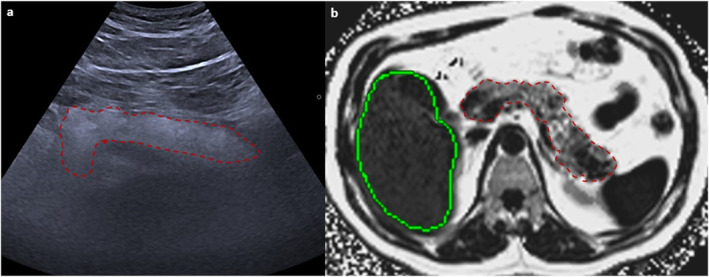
Transabdominal ultrasonography image (a) of a patient with fatty pancreas showing increased echogenicity, which was confirmed via magnetic resonance imaging‐proton density fat fraction (b).
